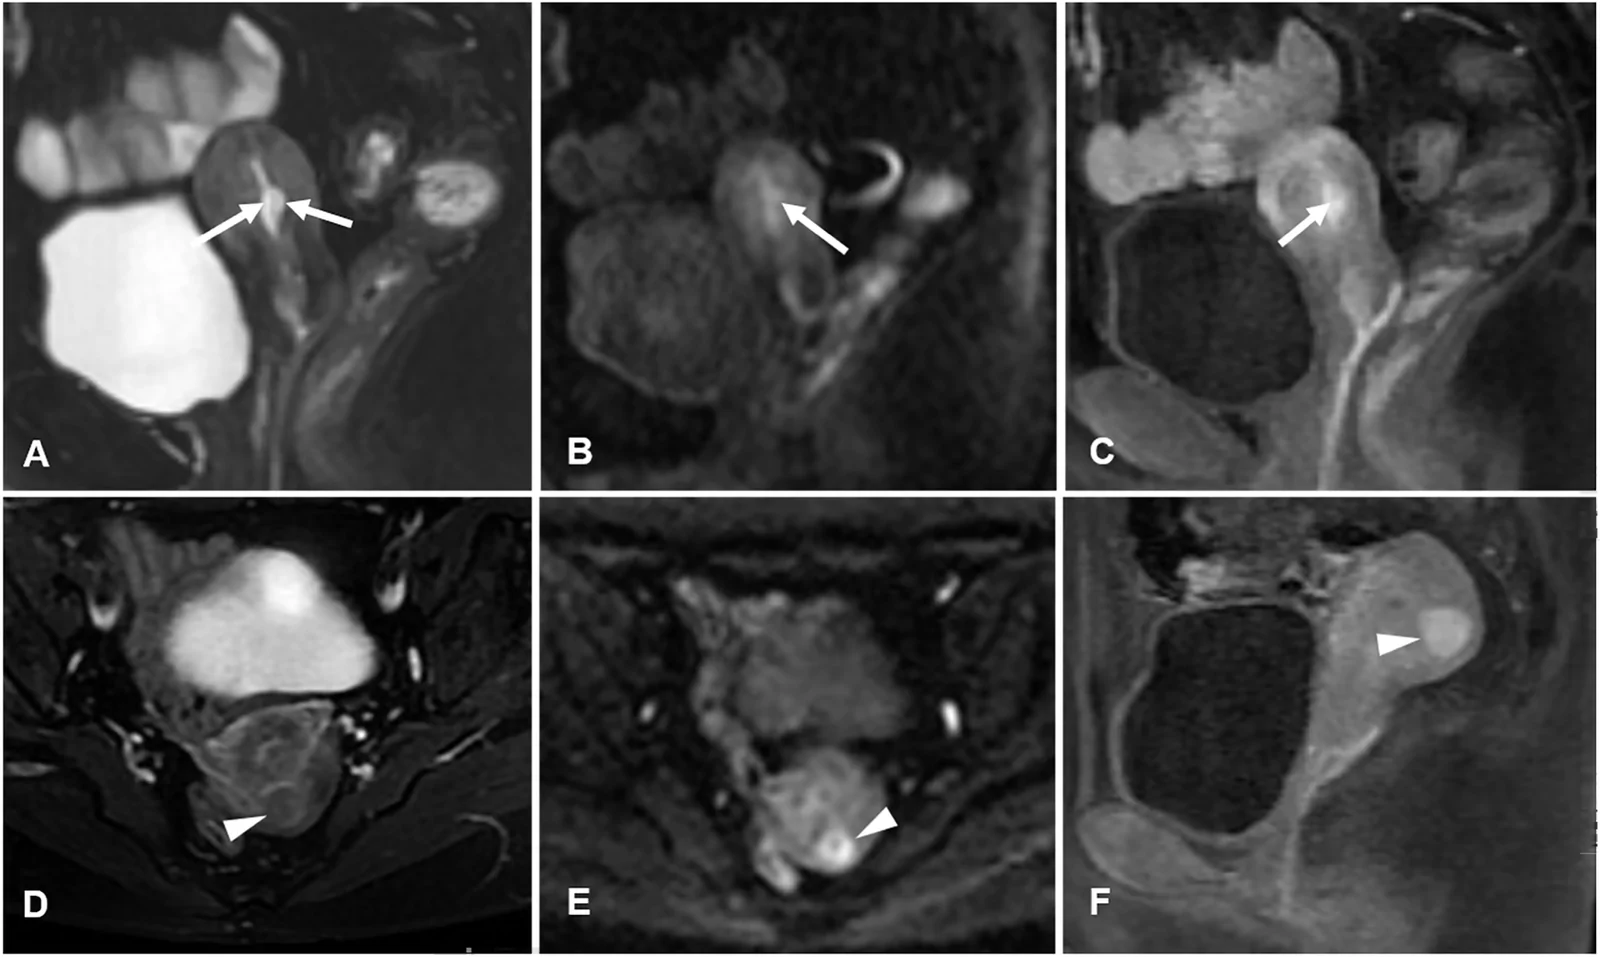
Magnetic resonance images of ESNs in a 63-year-old woman (A–C) and a 53-year-old woman (D–F). Sagittal T2WI (A) shows a lesion (arrows) located within the endometrial cavity, which has high signal intensity. Sagittal DW image (B) shows the lesion (arrow) with high signal intensity and ill-defined margins. Sagittal contrast-enhanced T1-WI (C) shows the lesion (arrow) with marked enhancement and well-defined margins. Axial T2W image (D) showing a lesion (arrowheads) located within the posterior myometrial wall, which is mildly hypointense (it is pathologically proven to be an ESN with smooth muscle differentiation). Axial DW image (E) showing a lesion (arrowheads) with high signal intensity. Sagittal contrast-enhanced T1-WI (F) showing the lesion (arrowheads) with marked enhancement and well-defined margins
